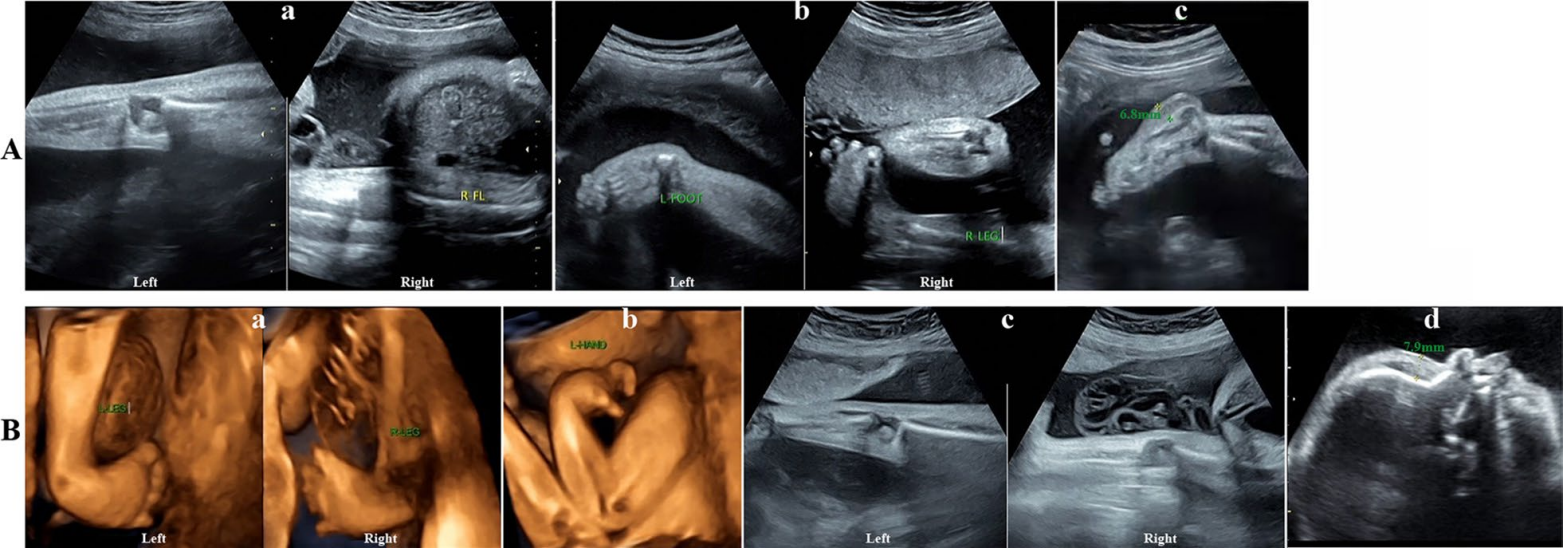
Ultrasound images. A Ultrasound images showing bilateral knee joint contractures (a), talipes equinovarus and inflexion (b), and increased thickness of planta skin (6.8 mm) (c) in patient 1. B Ultrasound images showing closed hands, flexed wrists (a), bilateral talipes equinovarus (b), extended legs and knee joint contractures (c), and thickening of the forehead soft tissue (7.9 mm) (d) in patient 3
